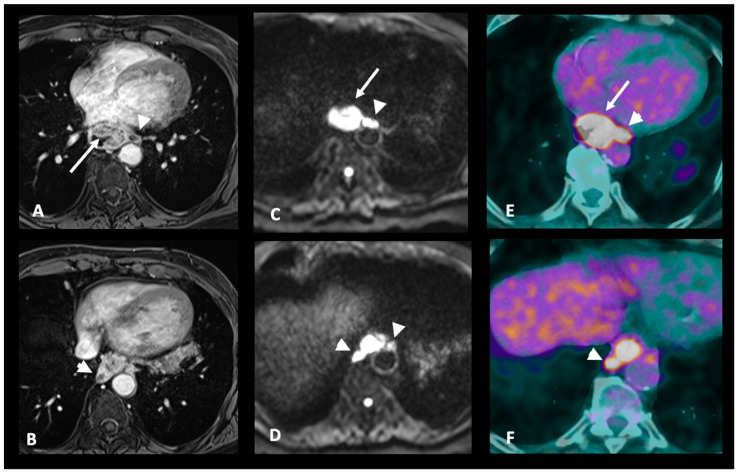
A 69-year-old patient presenting with an endoscopically unpassable tumor. The MRI images in T1WI FS GD (A,B) and DWI (C,D) show a large tumor in the lower third of the esophagus invading the periesophageal fat (white arrows). Multiple necrotic periesophageal lymph nodes are also present (arrowheads), classifying the tumor as MR-T3N2. The FDG PET/CT images (E,F) show intense tracer uptake at the esophageal tumor and periesophageal lymph nodes, confirming their tumoral nature. MRI Images acquired on a 3 T-scanner (Magnetom PrismaFit, Siemens Healthcare). WI: weighted imaging; DWI: diffusion-weighted imaging; and FS GD: fat sat with gadolinium.
